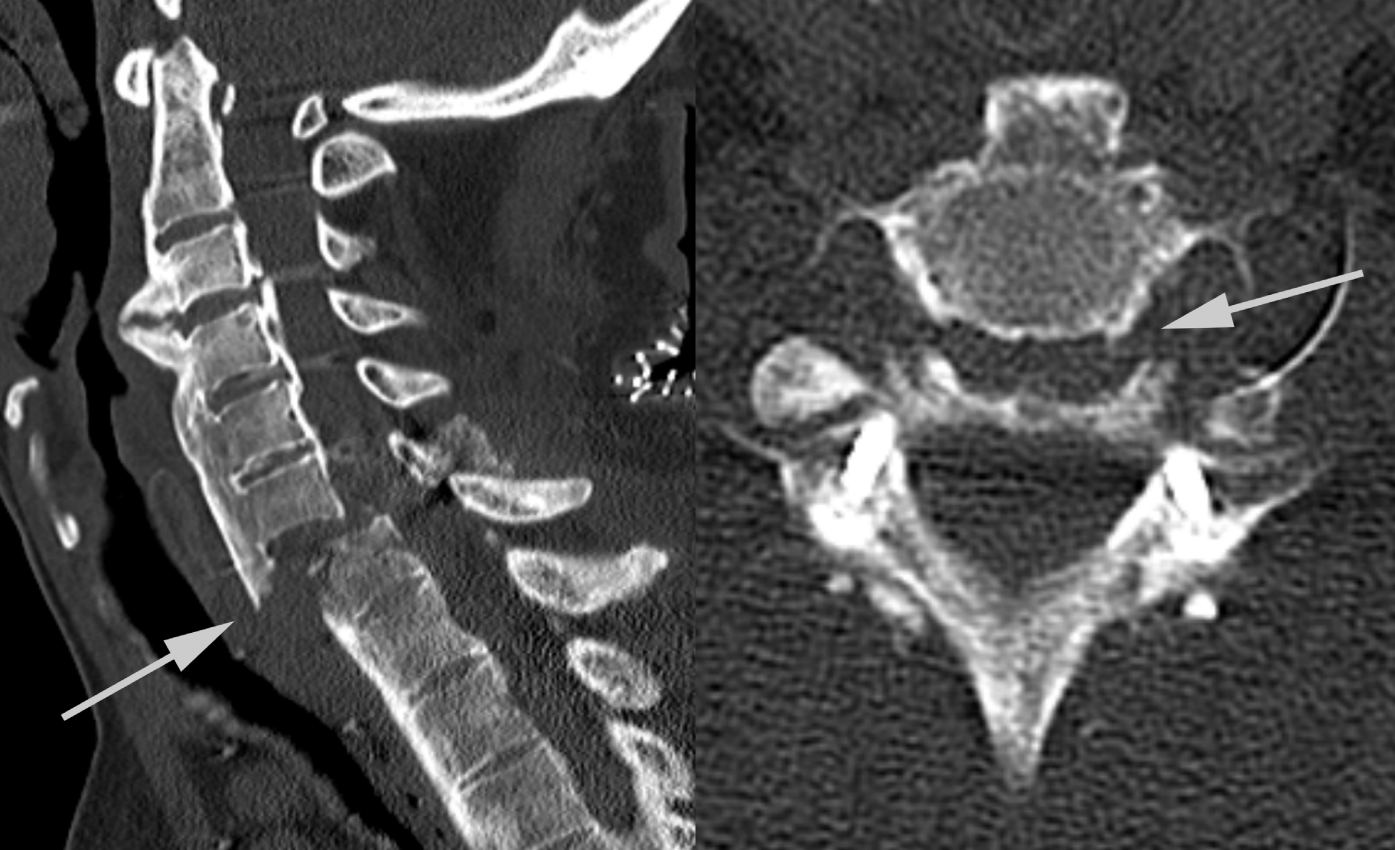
Computed tomography scan of our patient's cervical spine obtained on admission to our institution. The scan shows a persistent luxated position of the fracture at level C6/7 after dorsal stabilization at the outside hospital.
